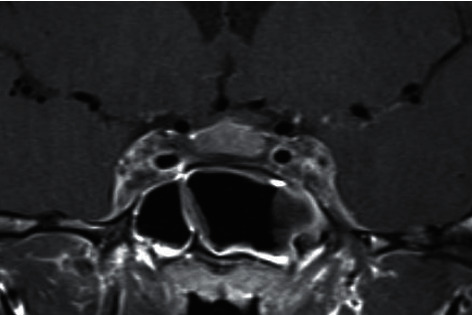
Coronal T1 postcontrast MRI demonstrates an enlarged and rounded appearance of the cavernous sinus with heterogeneous enhancement, consistent with cavernous sinus thrombosis.
